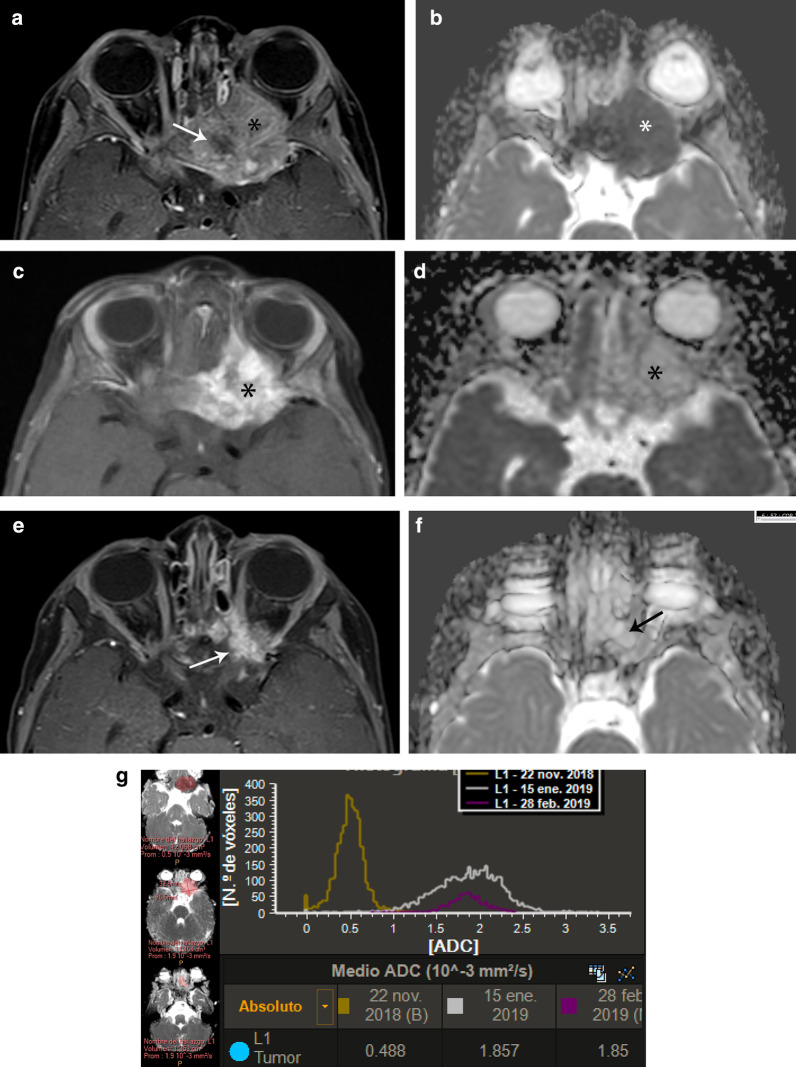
Ewing sarcoma involving the sphenoid bone in a 15-year-old boy. a Axial contrast-enhanced fat-suppressed T1-weighted MR image of the orbits shows a heterogeneously hyperintense mass (*) arising from the sphenoid bone with internal necrosis (arrow). b Axial ADC map shows restricted diffusion of the mass (*). c Axial contrast-enhanced fat-suppressed T1-weighted MR image performed after 2 cycles of chemotherapy shows decrease in volume of the mass with heterogeneous enhancement of the residual tumor (*). d Axial ADC maps shows no restriction of the residual mass (*). e Axial contrast-enhanced fat-suppressed T1-weighted MR image performed after 3 cycles of chemotherapy shows residual enhancing tissue (arrow). f Axial ADC map shows no restriction (arrow). g Volumetry of ADC maps performed before (yellow) during (white) and after (purple) treatment. Before chemotherapy, the tumor volume is 12 cc with corresponding high and sharp ADC (yellow) histogram of 0.49 mm^2/^s. During treatment, the volume does not significantly change (11 cc), but the corresponding volumetric ADC (white) histogram increases to 1.85 mm^2/^s, appearing short, wide and shifted to the right. After treatment, the volume decreases to 1.35 cc and the ADC (purple) histogram does not change (1.85 mm^2/^s). Overall, this indicates good treatment response
